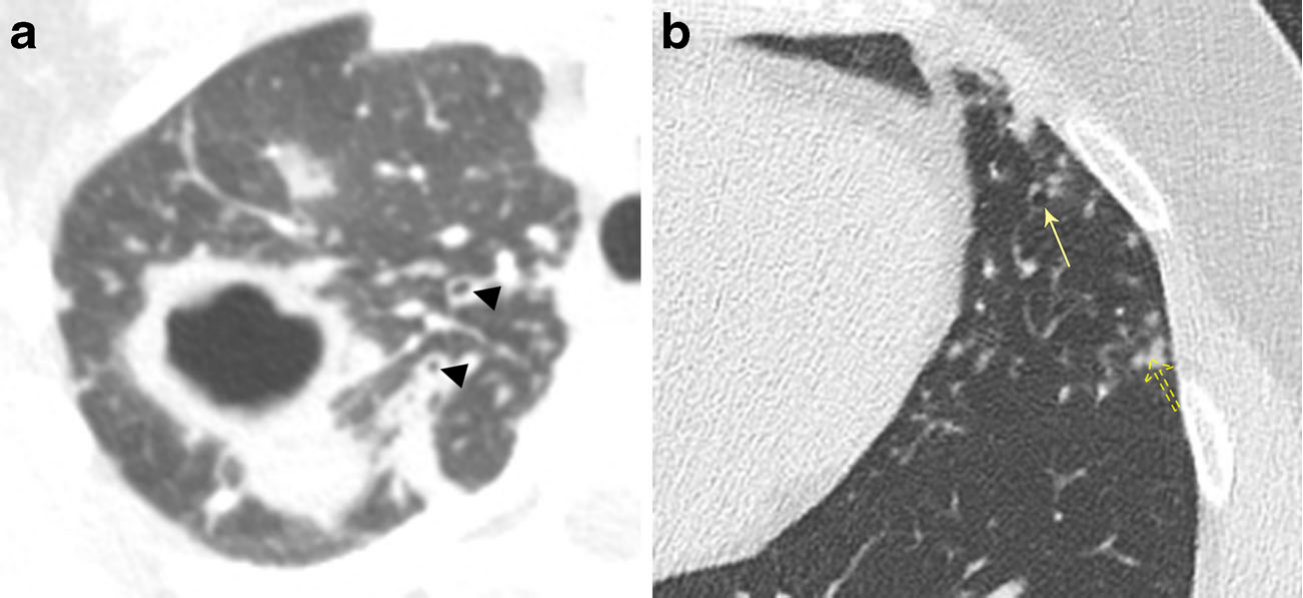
Bronchiectasis due to *Mycobacterial avium complex* (MAC) infection. a Axial CT imaging demonstrates an upper lobe-predominant cavitary process typical of the classic form of MAC infection with associated areas of bronchial wall thickening and bronchiectasis (*arrowhead*). b Axial CT imaging demonstrates the nonclassical form of MAC infection, also known as Lady Windermere syndrome, characterised by mild to moderate cylindrical bronchiectasis (*solid arrow*) typically affecting the anterior basal right middle lobe and lingula, often with associated ill-defined tree-in-bud or centrilobular nodules (*dashed arrows*)
